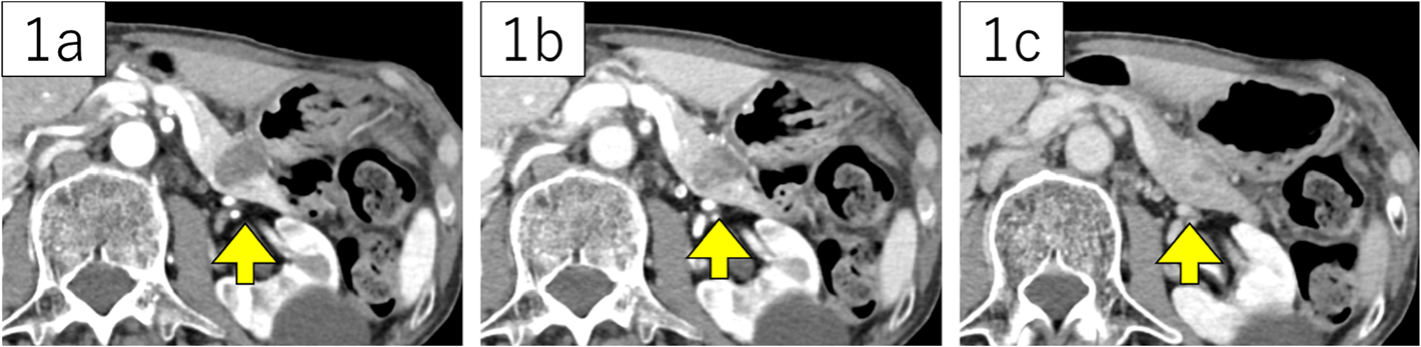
Dynamic computed tomography (CT) showed hypodensity in the arterial phase (1a), slight enhancement in the portal phase and iso- to hyper-density in the equilibrium phase (1b), compared to the parenchyma of the pancreas (1c), each indicated with arrowheads
